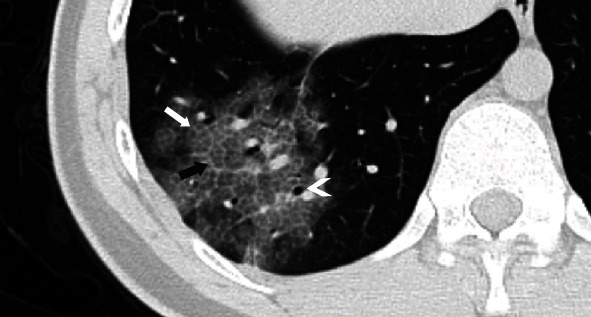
A 16-year-old boy presented with fever for 5 days. The axial CT image shows patchy ground-glass opacities with interlobular (black arrow) and intralobular (white arrow) septum thickening, showing a crazy-paving pattern. Bronchiectasis is also manifested (arrowhead).
